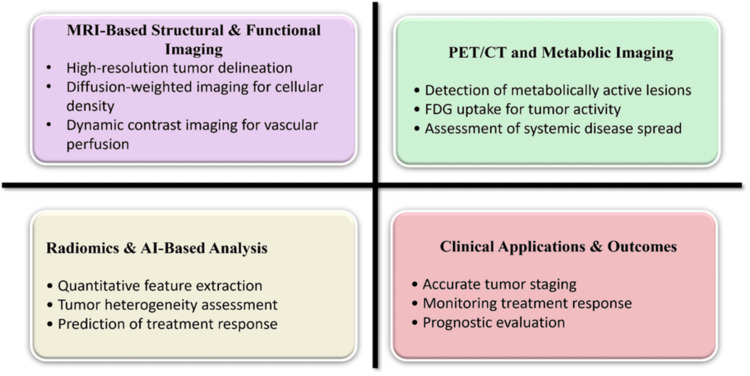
Multimodal imaging and AI integration in tumour evaluation Created by authors using MS PowerPoint (Microsoft, Redmond, WA) FDG - fluorodeoxyglucose
